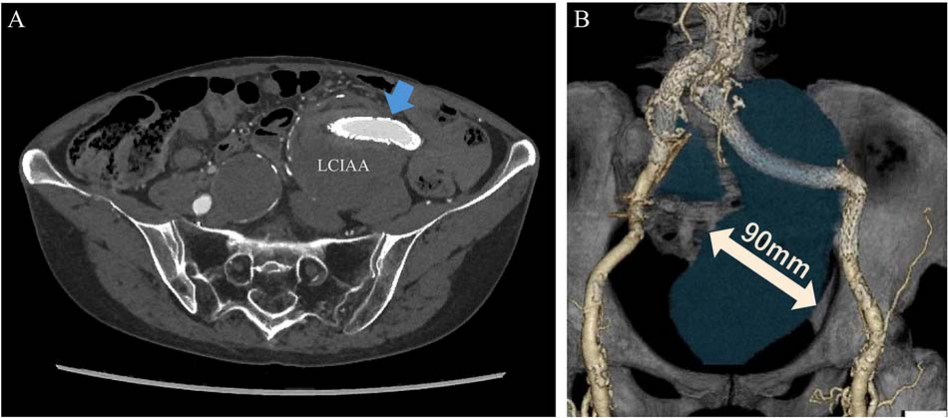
Preoperative computed tomography imaging showing a left common iliac artery aneurysm and internal iliac artery aneurysm with a maximum short diameter of 90 mm. A—Axial image, B—3D image. LCIAA, left common iliac artery aneurysm.
